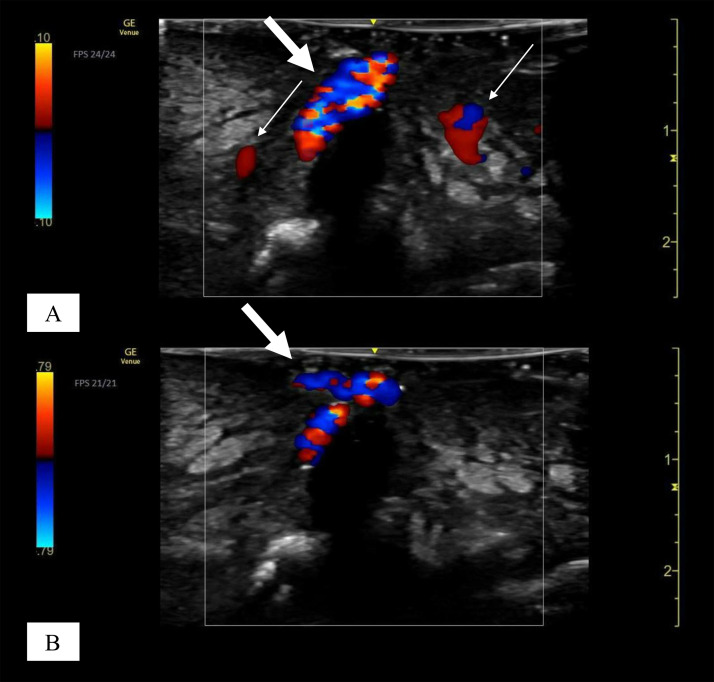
Colour Doppler images of larvae. (A) The larval structure exhibited a sonographic twinkle sign (thick white arrow), and blood vessels demonstrated flow signals (thin white arrow) at a Doppler setting of 10 cm/s. (B) At a setting of 79 cm/s, no vascular flow was detected, while the twinkle artefact persisted (thick white arrow).
